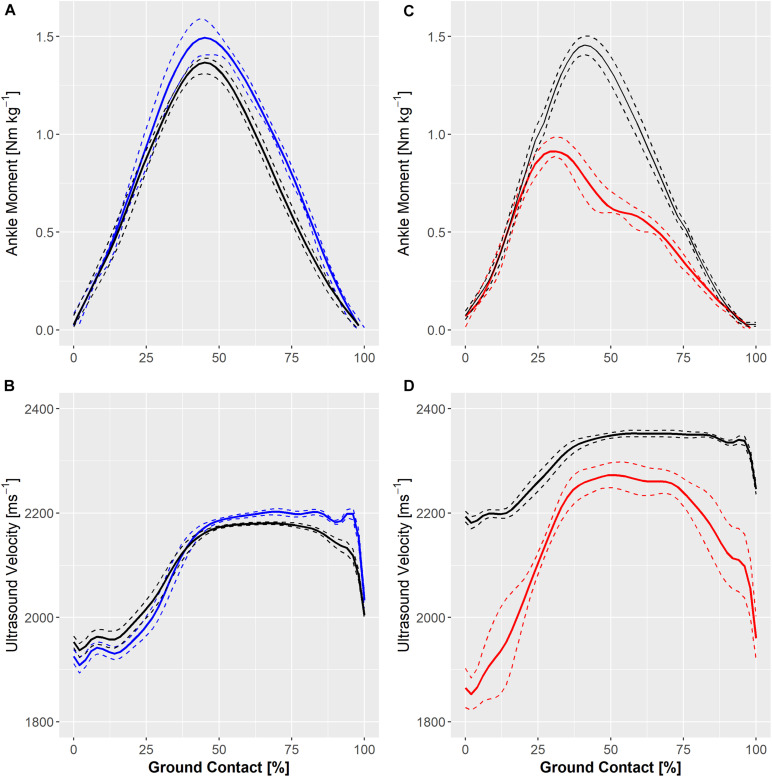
Individual ensemble histories for external ankle moments (upper panels) and ultrasound velocity in the Achilles tendon (lower panels) of a healthy adult during hopping at 1.8 Hz (solid blue line) and 2.5 Hz (A,B) and in an adult with a surgically repaired Achilles tendon (solid red line) and a matched healthy control hopping at 2.5 Hz (C,D). Standard deviations are represented by dashed lines.
